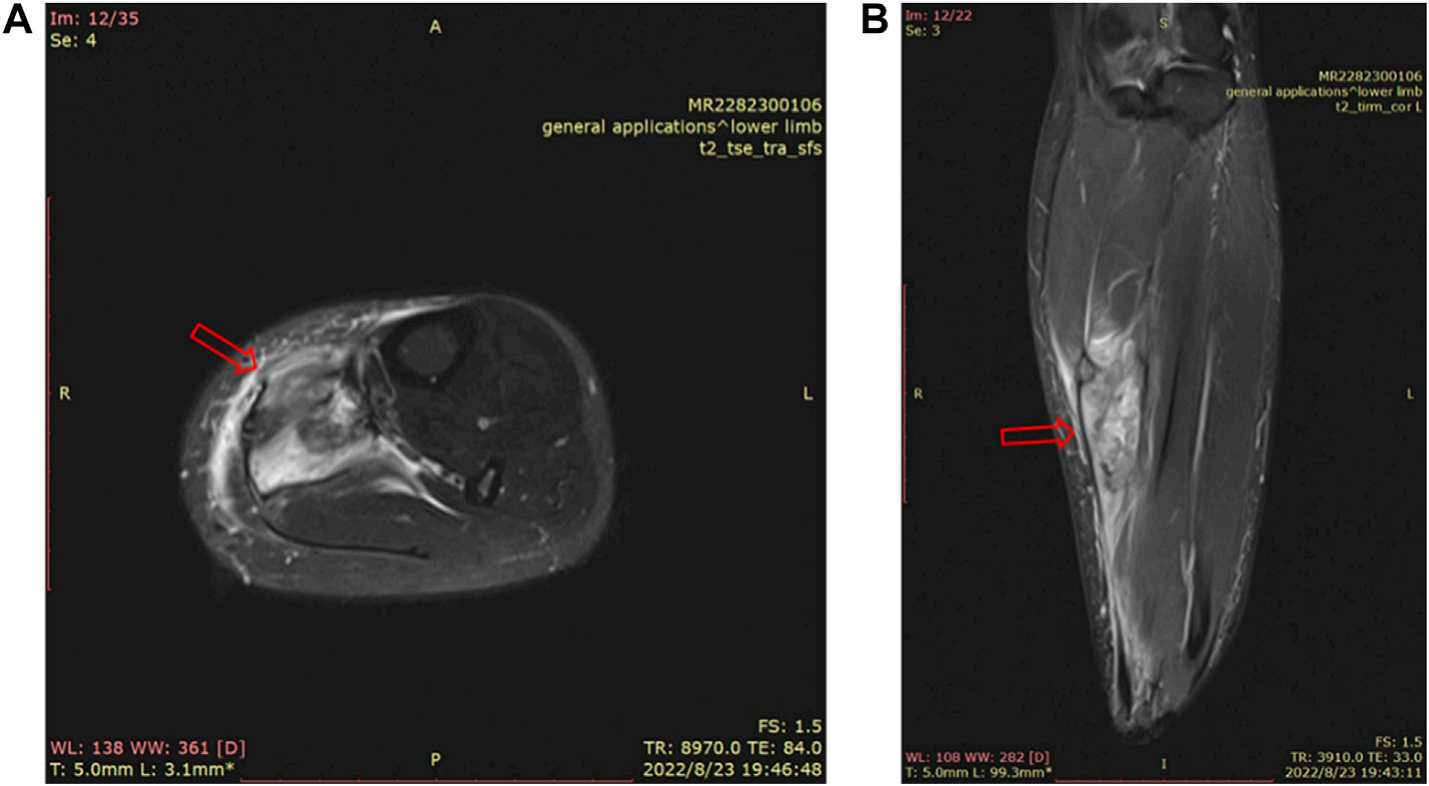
MRI of a 35-year-old female’s left calf. There is a mass-like high signal shadow in the flounder and posterior tibial muscles, a small amount of fluid in the intermuscular space, thickening and swelling of the surrounding fascia, and edema in the subcutaneous fat layer (A). MRI image of the cross section of the left calf. (B). MRI image of the sagittal plane of the left calf.
